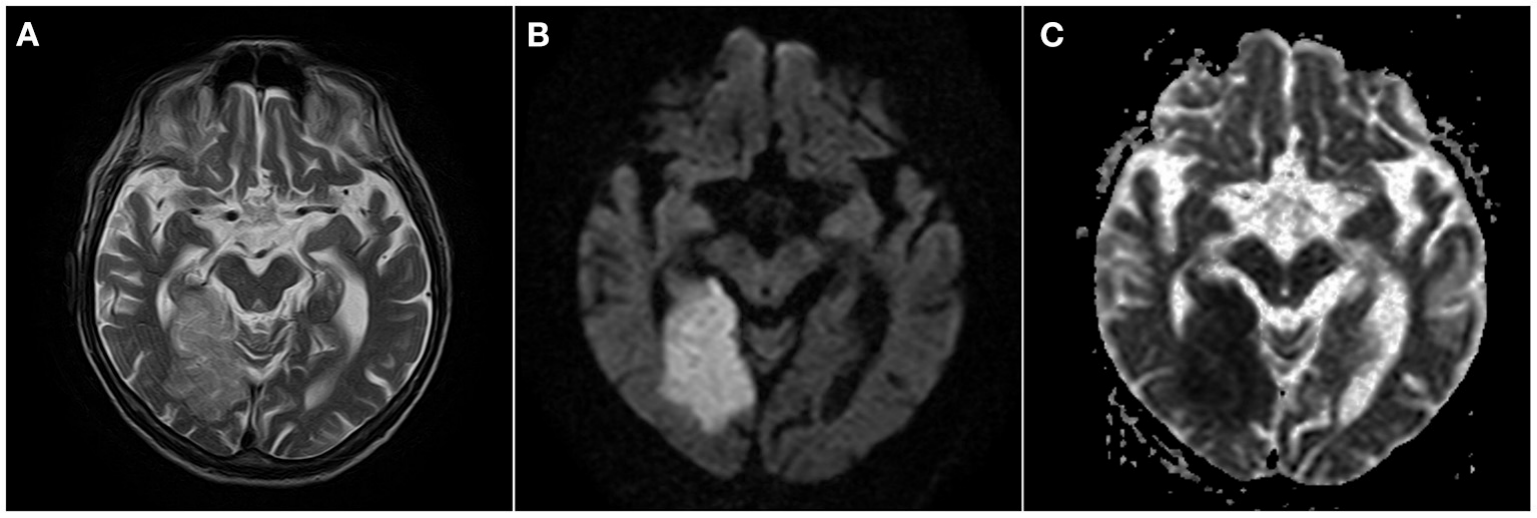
Acute ischemic stroke in the right occipital lobe. Hyperintense area of edema is seen on T2-weighted image (A). Diffusion restriction seen as marked hyperintensity on DWI (B) and reduced ADC values (C).
